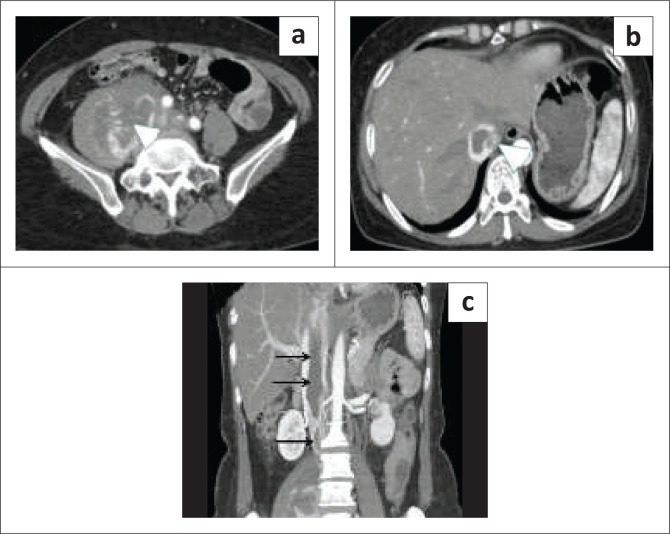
(a) Heterogeneous enhancing mass in the pelvic retroperitoneum (arrowhead) involving the psoas muscle, diagnosed as a sarcoma. (b) Intraluminal tumour thrombus extending up to the intrahepatic inferior vena cava (IVC) (arrowhead). In contrast to the bland thrombus, the tumour thrombus component shows enhancement. (c) The coronal CT shows the extent of IVC thrombus (black arrows).
